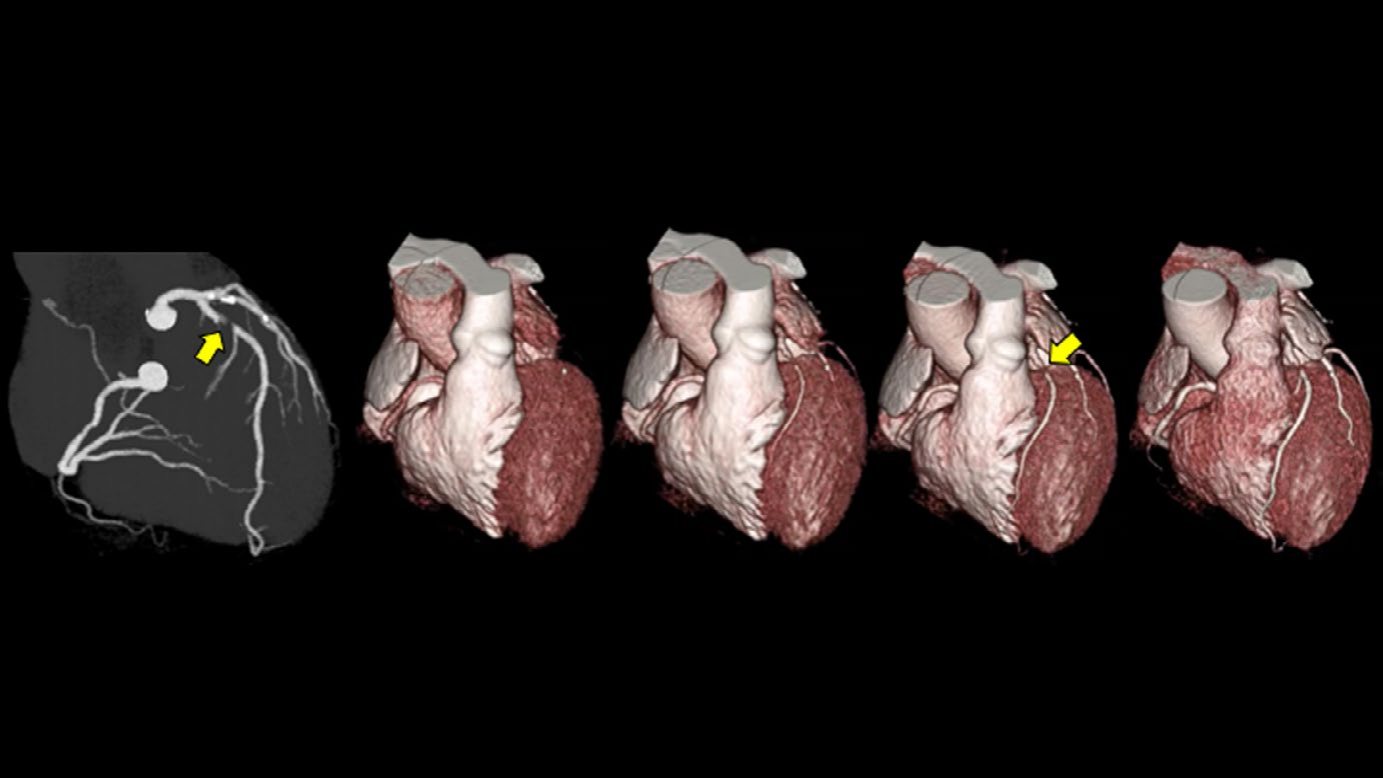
Angiographic view during the boost scan (left) and whole‐heart volume‐rendering images obtained from dynamic CCTA for a man in his 60s with severe stenosis at the proximal left anterior descending artery (arrows). Dynamic volume‐rendering images demonstrate the first pass of contrast medium from the heart cavities into their coronary arteries. Flow is traveling from the left to right over time. CCTA: coronary computed tomography angiography
